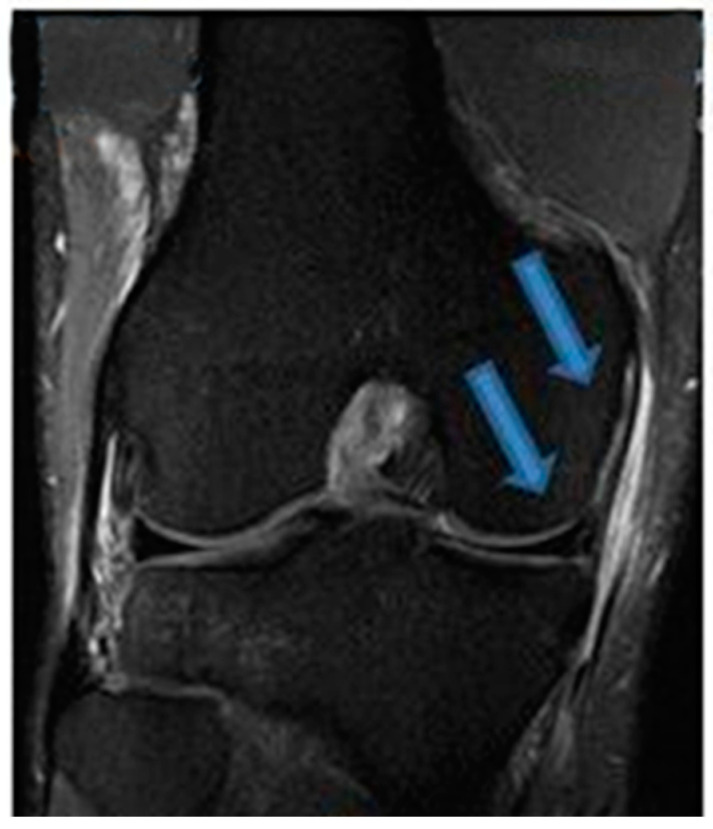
Coronal T2-weighted magnetic resonance imaging of the knee demonstrating injury of the medial meniscus and MCL indicated by blue arrows (20 July 2020).
